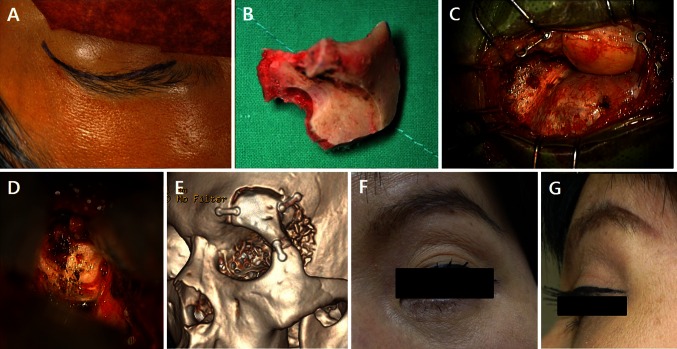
Surgical images. a Trans-eyebrow skin incision. b The bone flap. c The operation field. d Exposure of the petrous bone. e Postoperative bone-surface CT image. f, g Front and lateral view of the skin wound 3 months after surgery
